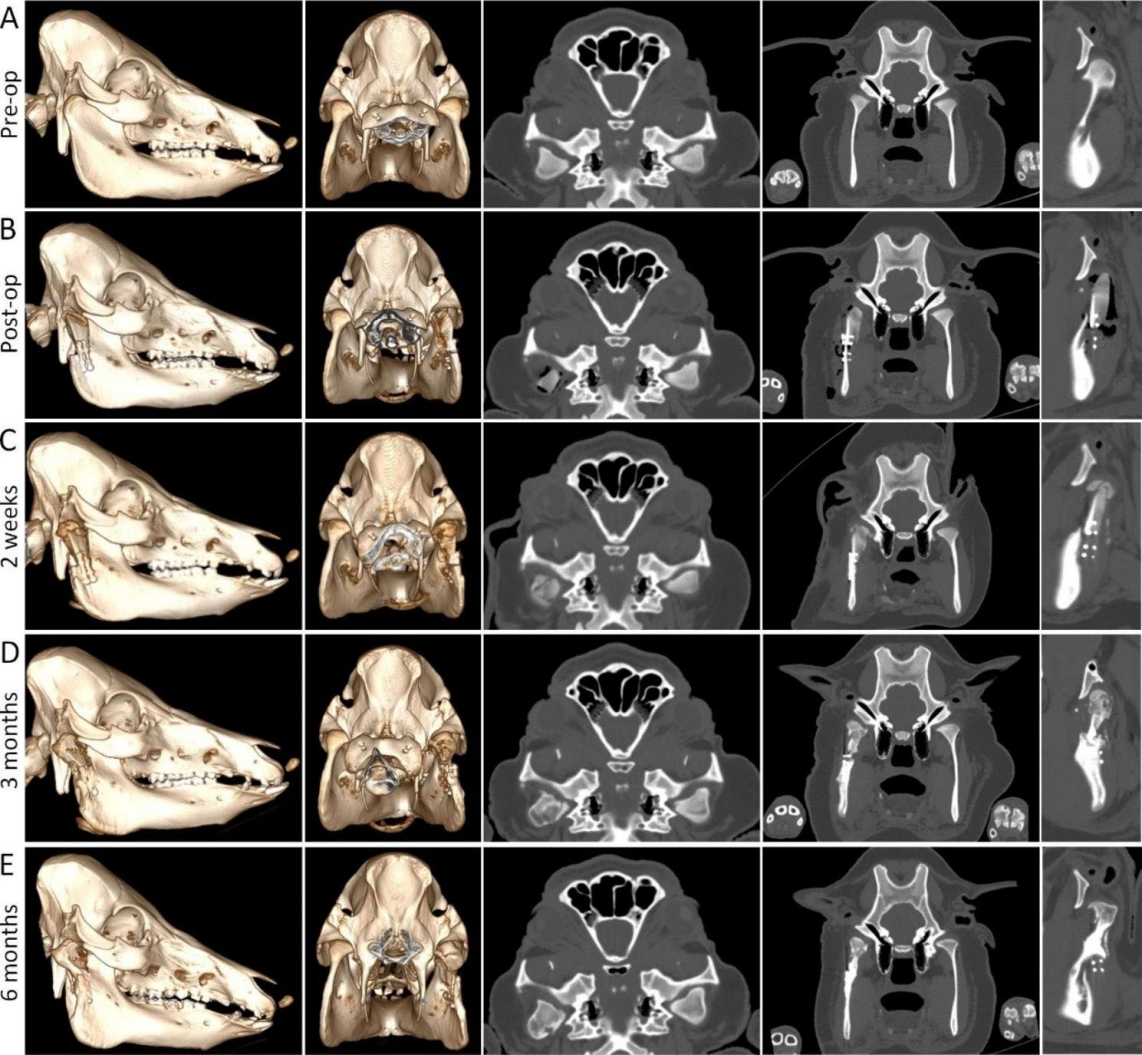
CT imaging at different stages. CT images acquired before (A), immediately after the operation (B), and at 2 weeks (C), 3 months (D) and 6 months (E) after the operation
